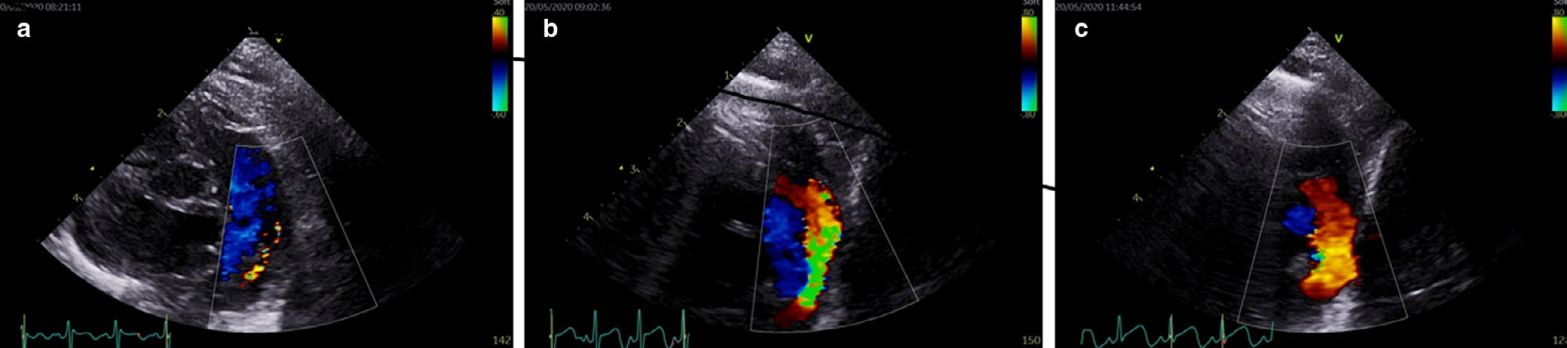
Flow through ductus arteriosus on color Doppler: Baseline (a), 100% oxygen (b), 20 ppm inhaled nitric oxide (C)
